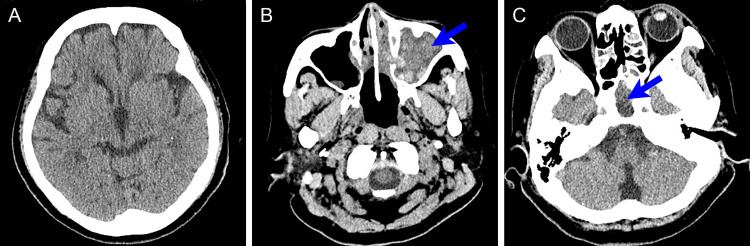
Head CT scan obtained 1 month prior to the ictus when the patient complained of a chronic headache (A) Plain head CT scan at the foramen of Monro level shows no abnormal findings. (B, C) Plain head CT scan at the parasinus level shows maxillary (B) and sphenoid sinusitis (C) (arrows), with mixed high- and low-density areas. No bony destruction is observed. CT: computed tomography
